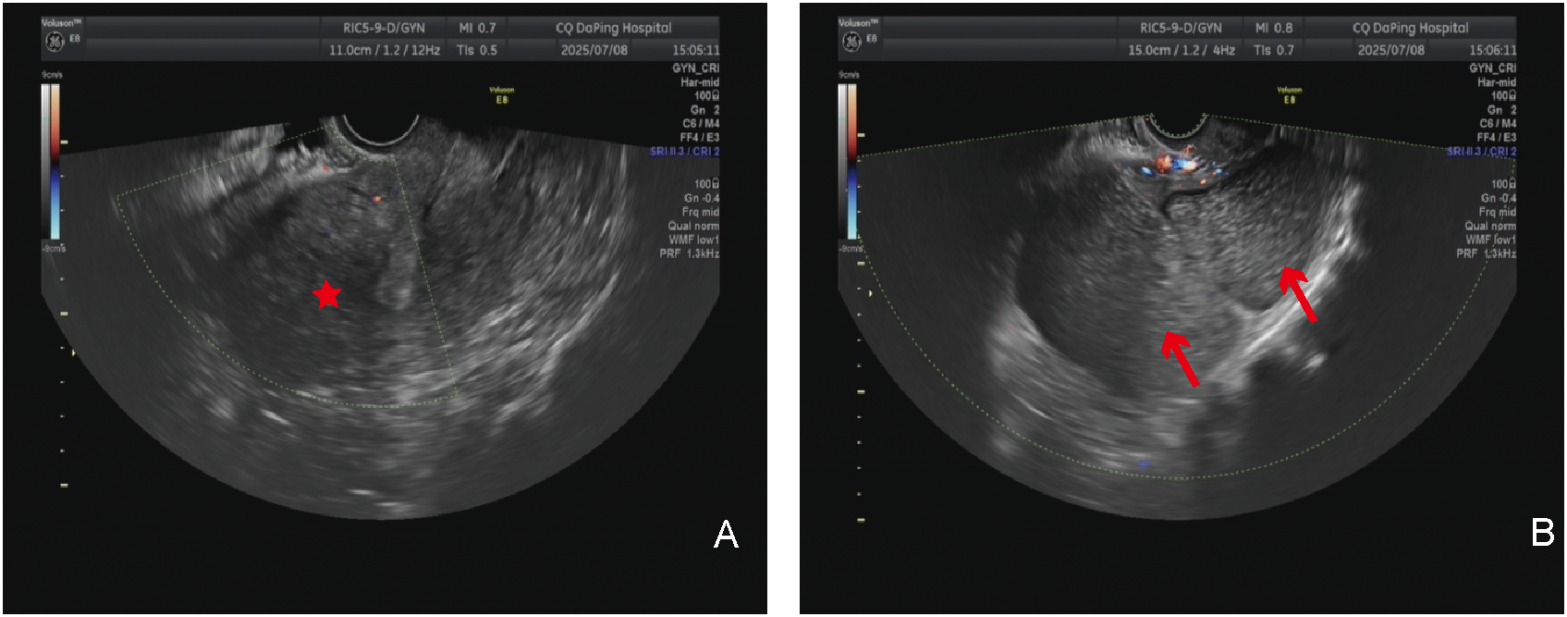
Imaging examination: (A) Transvaginal ultrasound examination; the red asterisk indicates the uterus. (B) Transvaginal ultrasound examination; the red arrow shows the giant cyst of the uterus.
